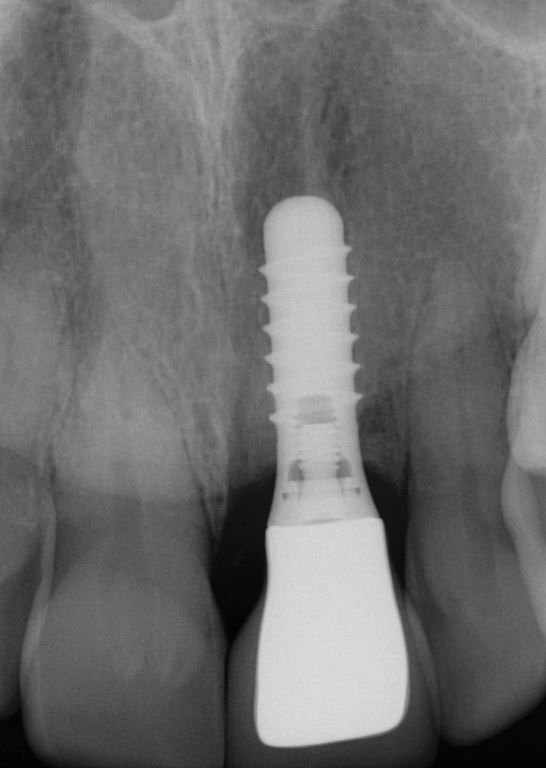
Intraoral periapical radiograph six months post debridement showing apparent radiographic bone infill
